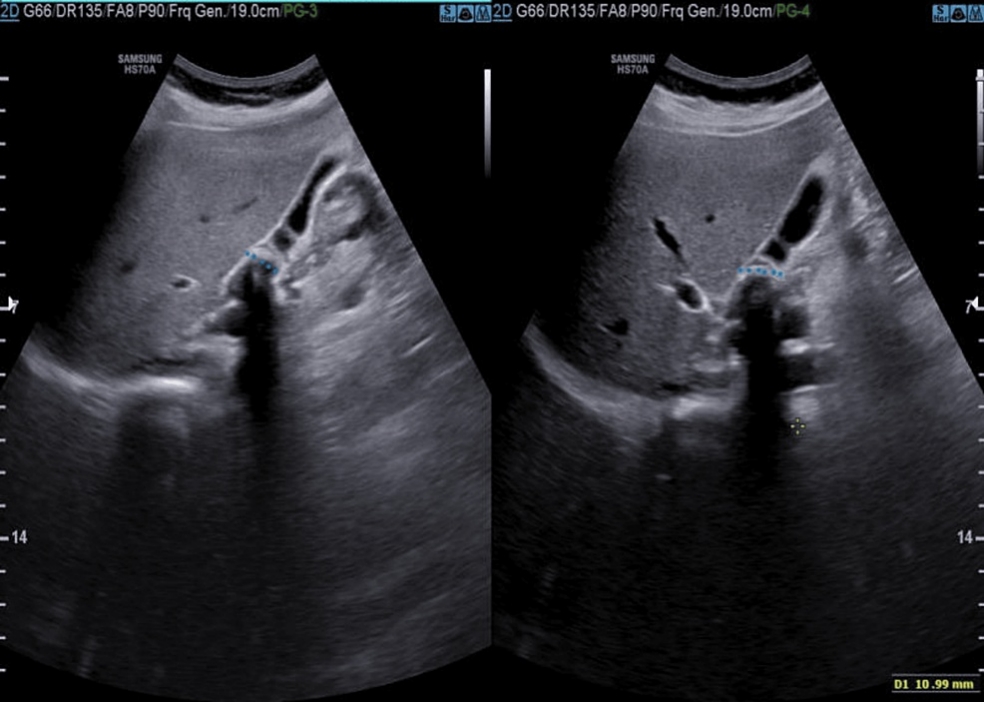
Ultrasound of the abdomen Gall bladder partially contracted with thick and edematous wall and showing 1.4 cm calculus impacted at the neck.
